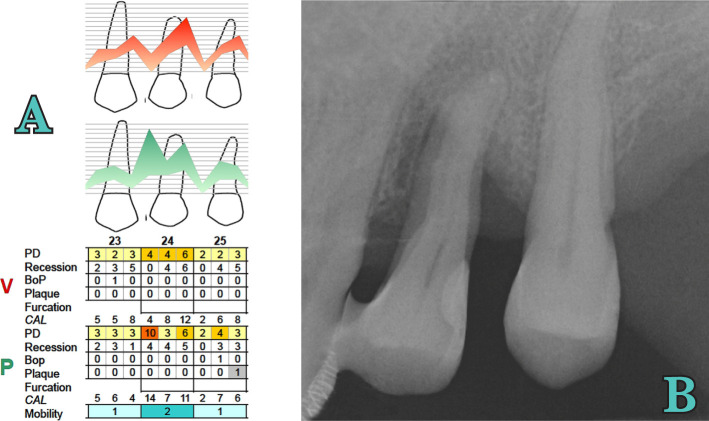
X-ray at 3 months from the initial examination - periodontal (A) and radiographic examination (B).
